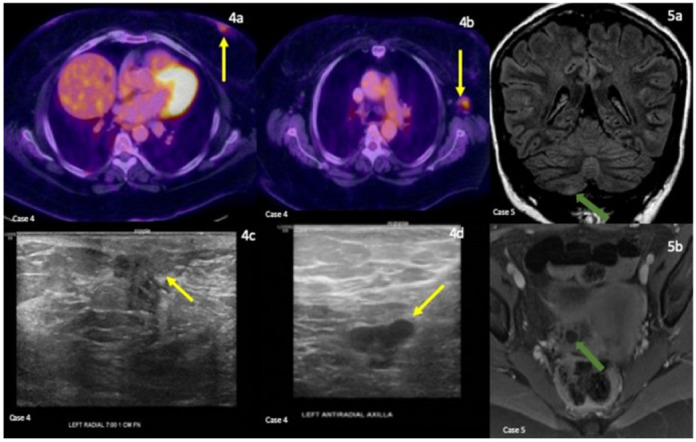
CASE 4: PET/CT of the chest (4a,4b), breast (4c), and axilla (4d) ultrasonography. Left breast subareolar hypermetabolic mass (4a) with hypermetabolic left axillary lymphadenopathy (4b) corresponding to suspicious left breast mass (4c) and left axillary lymph node (4d) on ultrasound. Coronal FLAIR (5a) sequence. Focal FLAIR hyperintensity in the right inferomedial cerebellum. Pelvic MRI with axial T1 fat suppressed post contrast (5b) sequences. Non-enhancing intrinsically T1 hyperintense right ovarian mass demonstrating fat suppression consistent with a teratoma.
